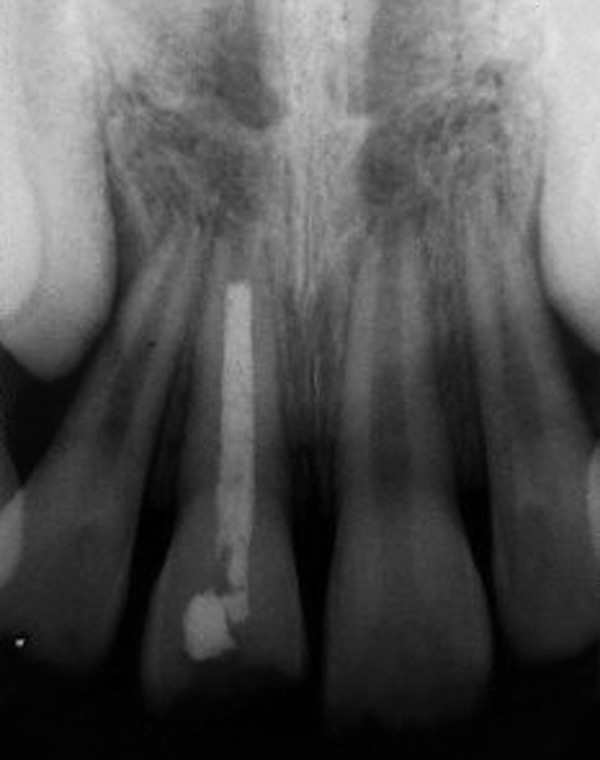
Preoperative intraoral periapical radiograph (case 1)
